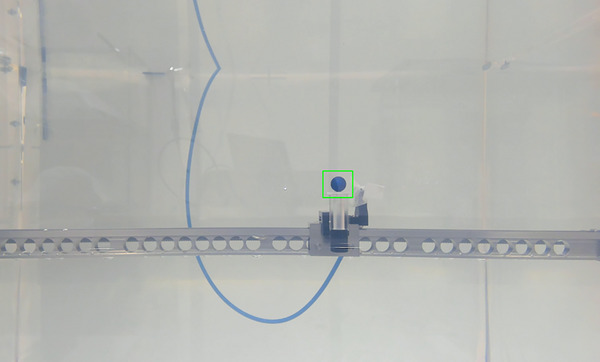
Measurement of the driving speed of the ET CT Body Marker using tracking software.
